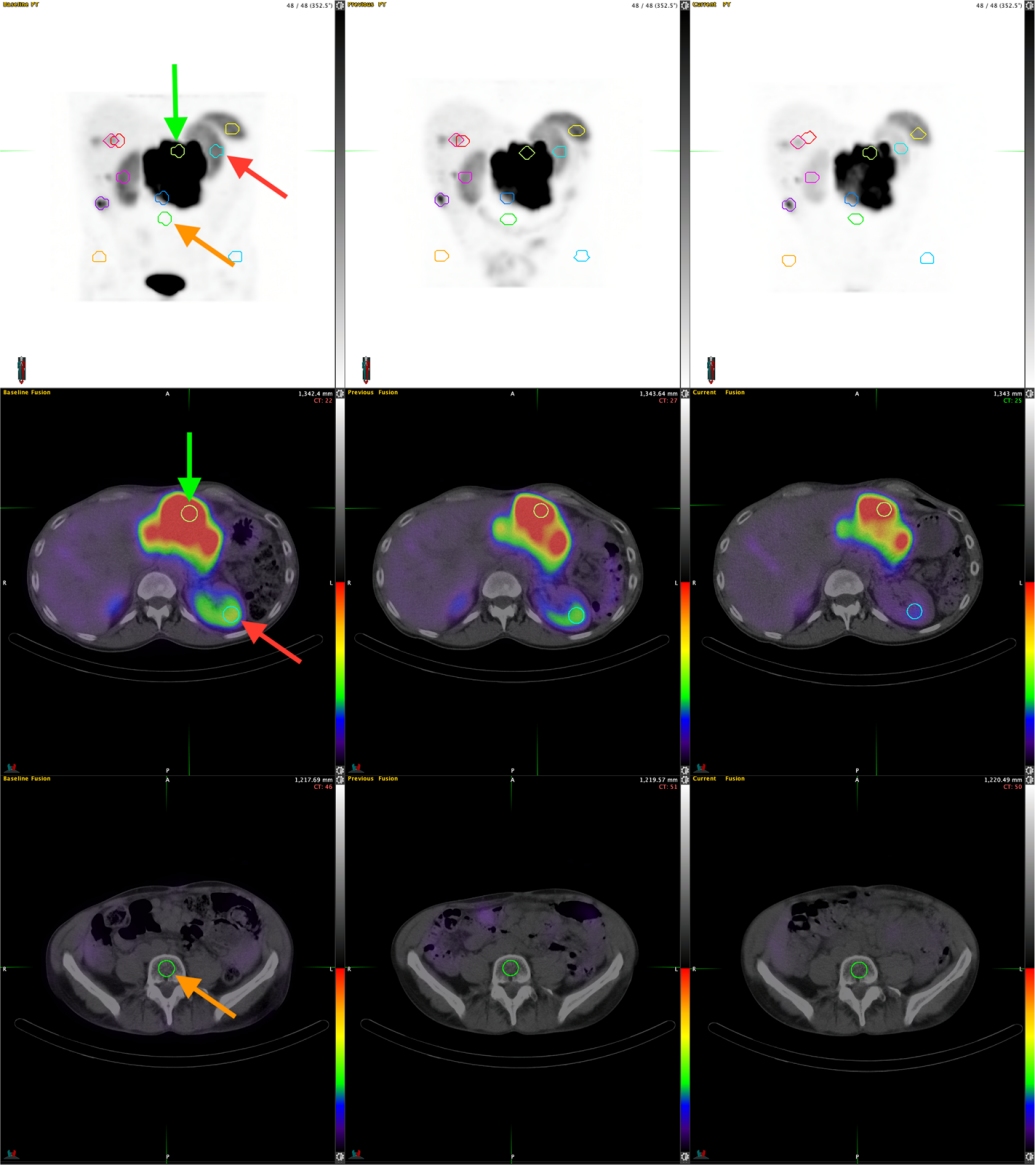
Post-treatment serial QSPECT/CT was performed at (from left to right) 5, 24, and 70 h after a 22.0 GBq ^177^Lu-octreotate administration in a 55-year-old male with metastatic NET of unknown origin. Small volumes of interest (2-cm diameter) were placed over tissues of interest. Left kidney (red arrows), L5 bone marrow cavity (orange arrows), and dominant tumor (green arrows) VOIs are pointed on anterior maximum intensity projections (top row) and selected transaxial fusion slices (mid and bottom rows). QSPECT images are normalized using an upper SUV threshold of 7. During this consolidation cycle, the personalized injected activity allowed the delivery of 6.1 Gy (6.0 Gy prescribed) to the kidney
